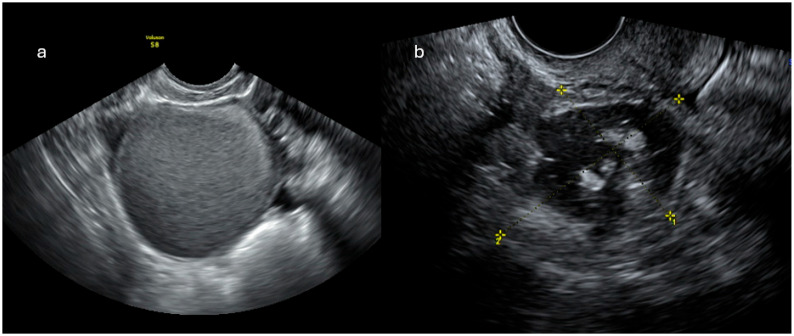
Ultrasound demonstrates a unilocular cyst with a “ground-glass” appearance, consistent with an endometrioma (a), and a lesion containing intralesional fat, typical of a dermoid (b); both representing classic benign ovarian lesions: O-RADS US score 2 (Courtesy of Dr. Gilda Di Paolo).
